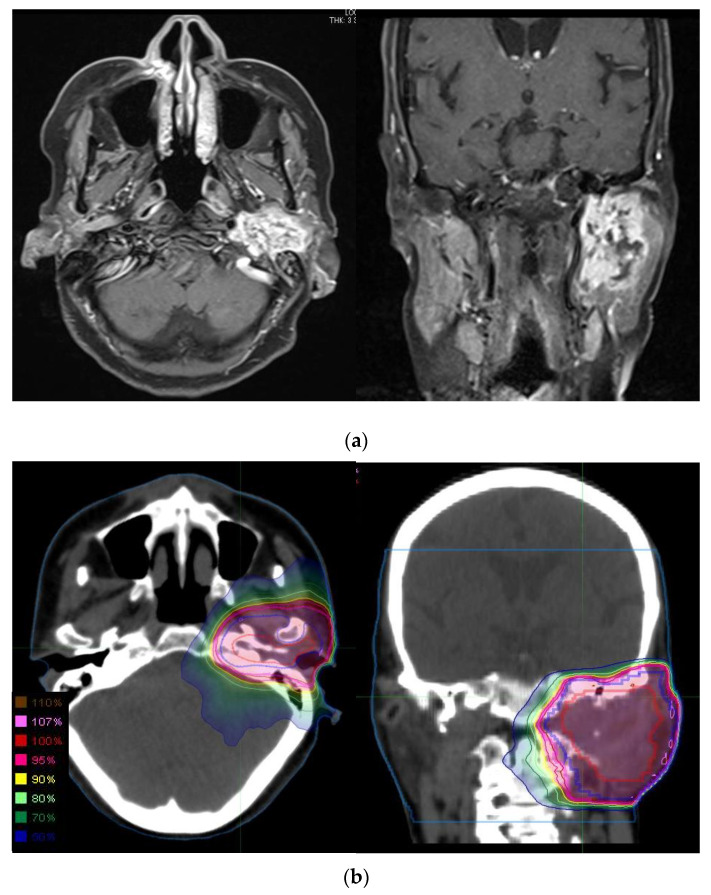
Representative case of parotid gland adenoid cystic carcinoma treated with defRT using IMRT. The 75-year-old woman with left parotid gland cancer, cT4bN0M0. Left facial nerve disorder was observed. (a) MRI contrast-enhanced T1-weighted images revealed the parotid gland tumor with the extension to base of skull. The patient refused surgery and hoped to receive defRT. (b) Dose distribution of defRT using 66 Gy in 33 fractions. The GTV, CTV, and PTV are shown in red, blue, and magenta, respectively. CTV margin was extended to base of the skull considering perineural invasion. PTV margin was added to CTV to account for patient motion and the field margins. Abbreviations: defRT, definitive radiotherapy; IMRT, intensity-modulated radiotherapy; MRI, magnetic resonance imaging; GTV, gross tumor volume; CTV, clinical target volume; and PTV, planning target volume.
